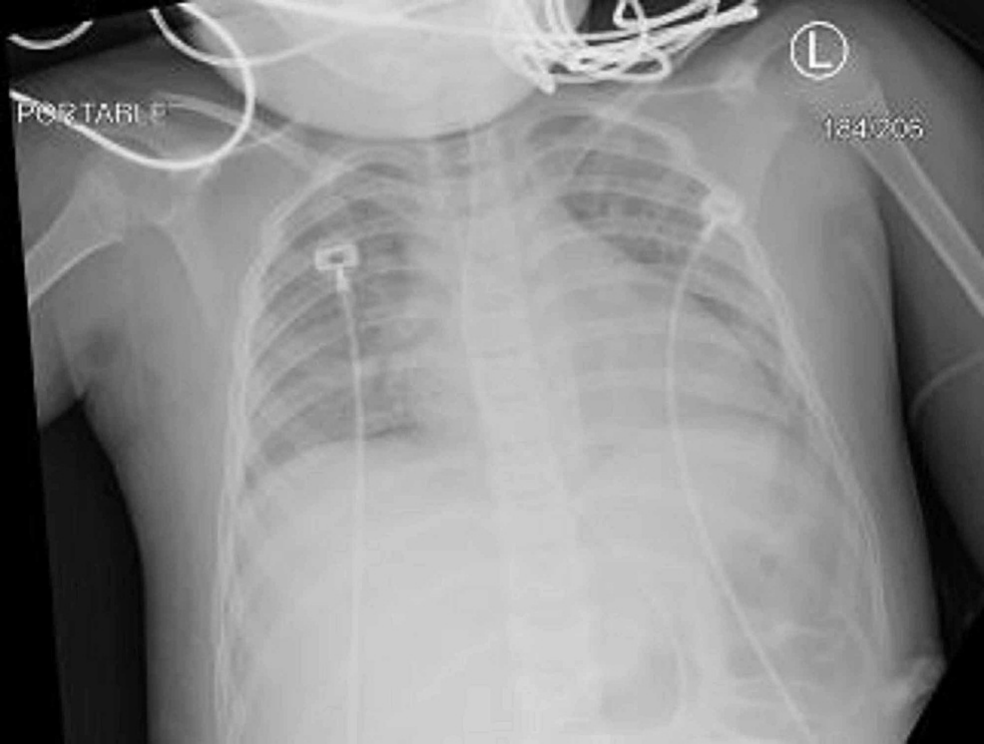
Chest x-ray post-extubation, showing signs of improved aeration with possible small airway inflammation as well as suspected small left effusion; however, assessment is limited by the low lung volumes.
